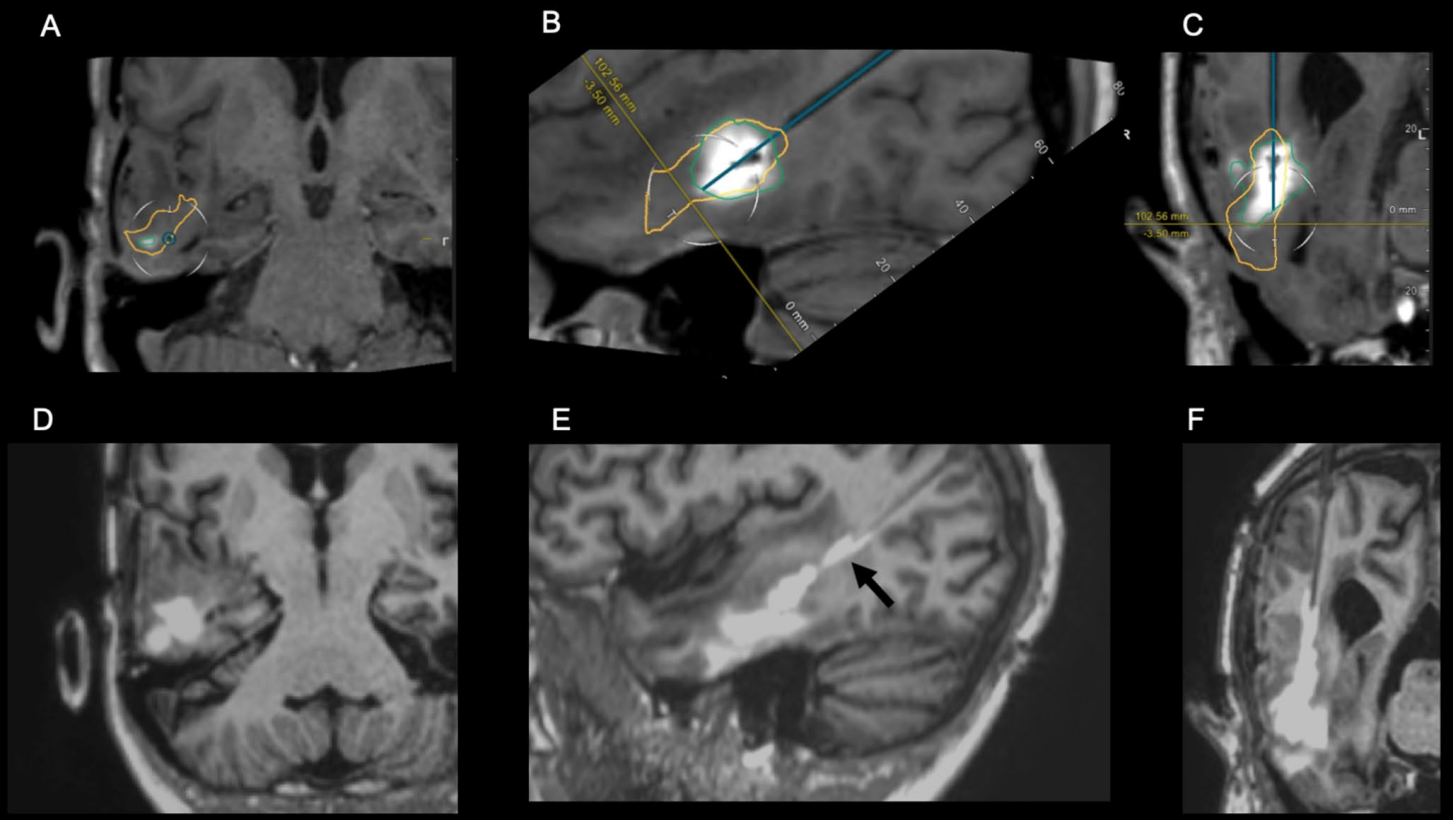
55 year-old patient with right temporal GBM recurrence (CED23). During CED, the area of gadolinium contrast deposition grows to cover 95% of the tumor. Images (A-C) show initial area of gadolinium contrast deposition on T1-weighted MPRAGE images in coronal oblique (A), sagittal oblique (B), and coronal oblique (C) planes, with green outline indicating the tumor and yellow outline indicating the final infusate coverage. Images (D-F) show final infusate coverage, with reflux of gadolinium contrast along the catheter tract (black arrow in E), that was seen during the procedure and allowed for correction of catheter trajectory
